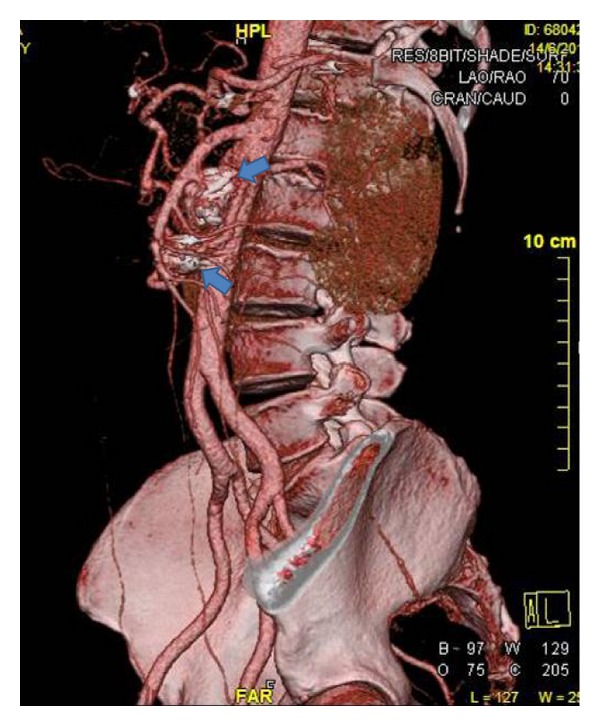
Follow-up imaging with volume-rendering lateral CTA views showing patent SMA and branches with thrombosis of both IPDA aneurysms and no sac reperfusion.
